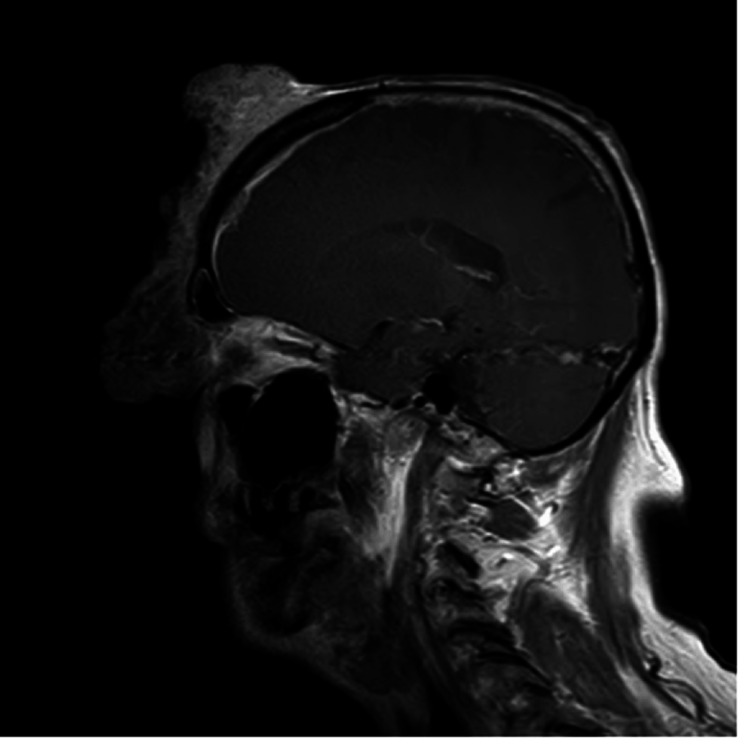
MRI face T1-weighted post contrast sagittal view of enhancing mass in the right frontal extra-axial space
